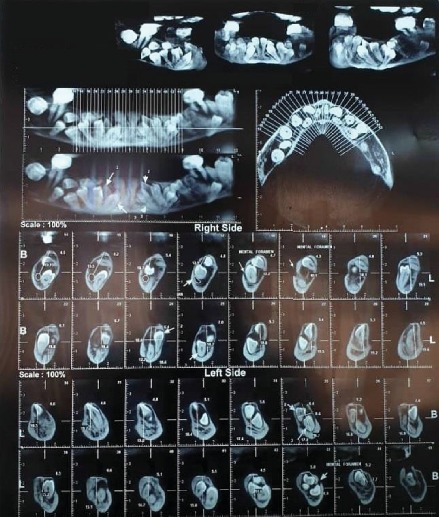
CBCT revealed 21 impacted teeth in mandible and 9 in maxilla.
